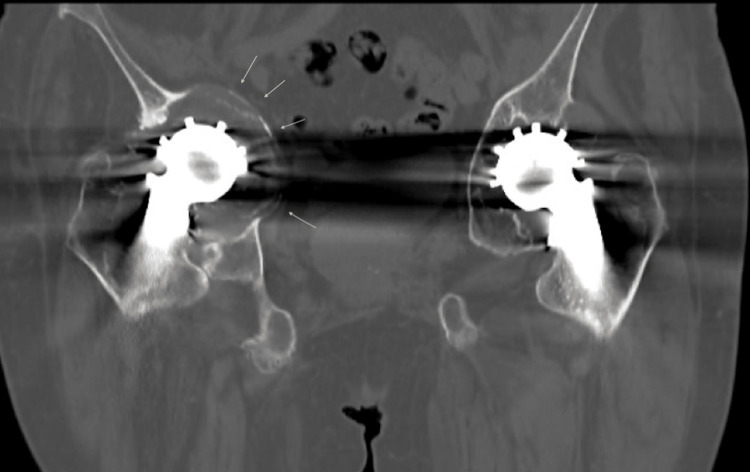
A coronal slice from the CT scan showing the extent of retro-acetabular osteolysis bilaterally, worse on the right side (see arrows)
